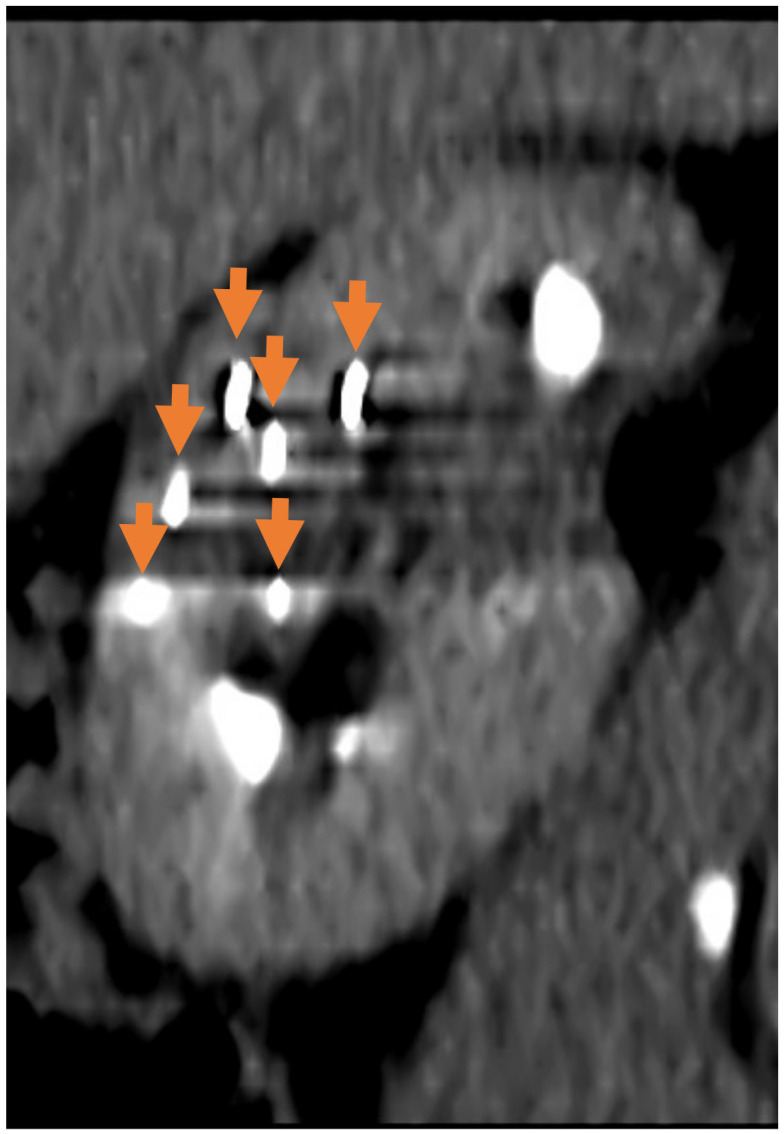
Intraoperative CT (sagittal view) showing 6 IRE needles (arrows) bracketing the tumour.
